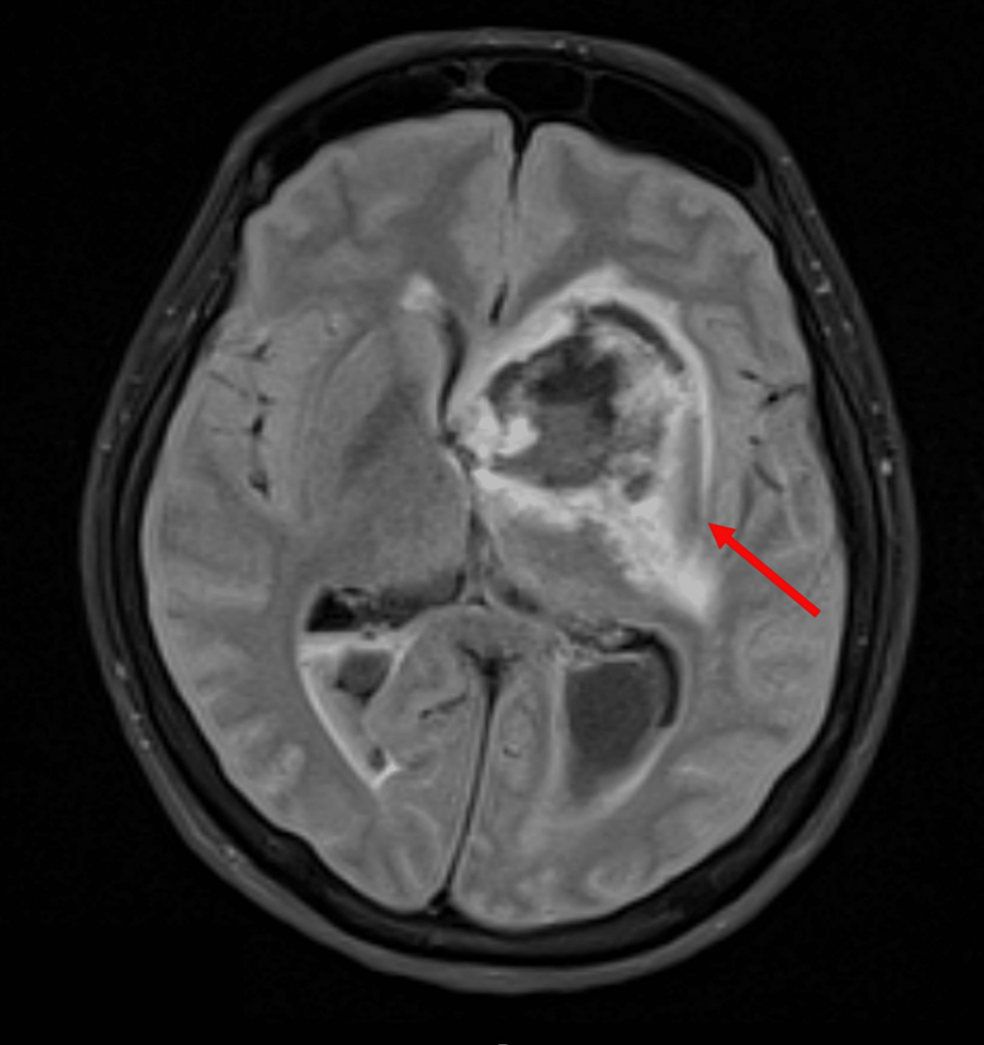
Brain MRI A neoplastic lesion (arrow), extending from the lenticulocapsular region to the medial aspect of the temporal lobe on the left, presenting perilesional oedema and a mass effect on the third ventricle and the left lateral ventricle, with a deviation of the median structures to the right
